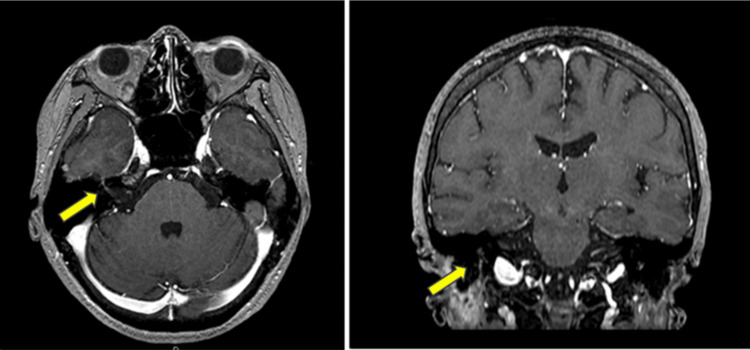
T1-weighted gadolinium-enhanced MRI image at the time of readmission The facial nerve running within the right temporal bone is enhanced compared to the left side (yellow arrow).
